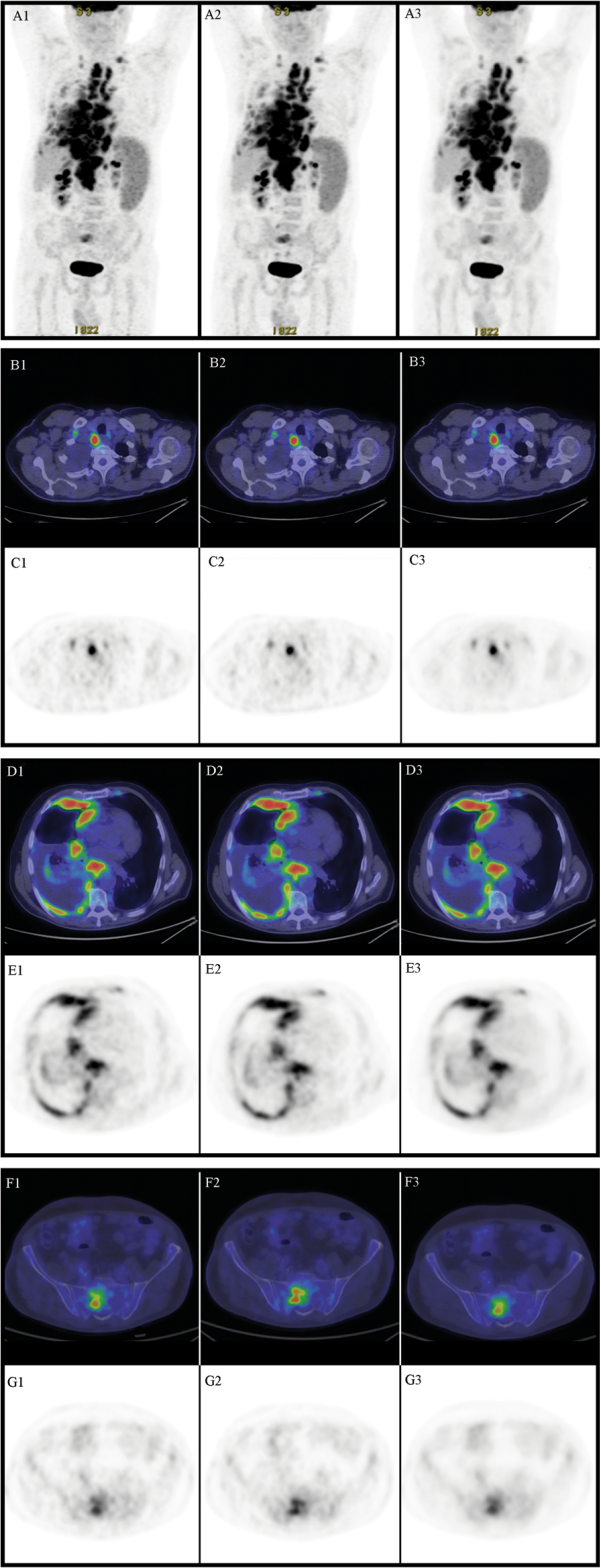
Staging in patient with pleural recurrence of mantle cell lymphoma. 1. Scan with reduced number of counts (acquisition with standard time and 66% FDG dose). 2. Native scan: scan with standard number of counts (acquisition with increased time). 3. Processed scan, resulting from the study with reduced counts (1) processed by AI SubtlePET^TM^. a Maximum intensity projection (MIP). b, d, f Fused transaxial positron emission tomography and computed tomography. c, e, g Transaxial positron emission tomography images. All images were visualized with AW server station by setting the same parameters (DFOV 57.8 × 35.8 cm, *M*=6.31 g/ml). The comparison between the studies shows a good quality of the processed by AI SubtlePET^TM^ images in the MIP visualization (Fig. 6a) and in the transaxial images, identifying the disease at the level of lymph nodes in the upper chest (Fig. 6b), at the level of the thorax (Fig. 6c) and at the sacrum (Fig. 6d)
